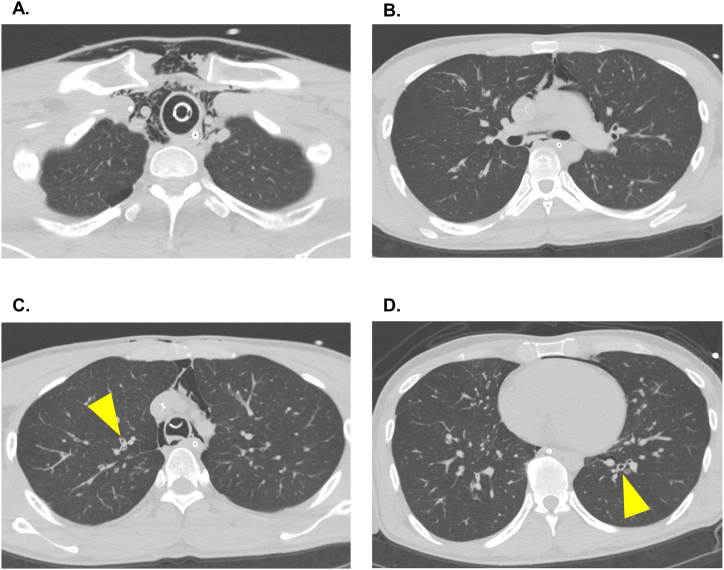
Chest computed tomography findings on admission before veno-venous extracorporeal membrane oxygenation (VV-ECMO) treatment (A) Mediastinal and subcutaneous emphysema due to invasive mechanical ventilation is observed. (B) Mediastinal emphysema due to invasive mechanical ventilation is observed. (C), (D) The arrow shows bronchial wall thickening.
